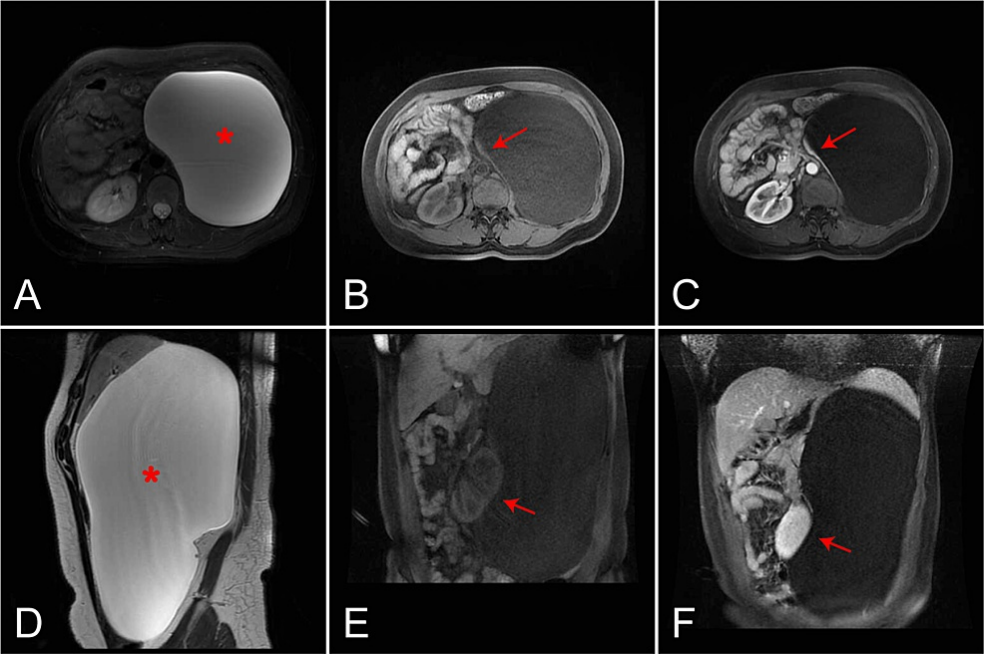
The imaging features of this cystic mass on magnetic resonance imaging. Axial (A) and Sagittal (D) T2-weighted MR images of the cystic mass demonstrate a predominantly high signal-intensity lesion (asterisk). Axial T1- (B) and enhanced T1-weighted (C) images show rim enhancement of the lesion, suggesting the left adrenal gland (arrows). Coronal T1- (E) and enhanced T1-weighted (F) images show the displaced kidney (arrows).
